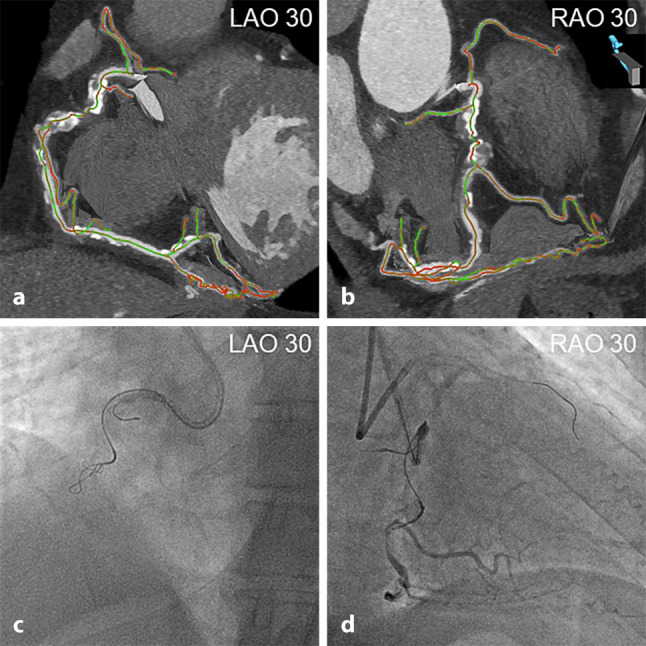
a–d Coronary computed tomography angiography (*CTA*) for guiding the knuckle wire technique. Colour-coded CTA multiplanar reconstructions demonstrating the exact course of a severely calcified and tortuous CTO of the right coronary artery (*RCA*) with clear delineation of the uptake of the right ventricular branch in right anterior oblique (*RAO*) 30° projection. The coronary segments are displayed in *green* (no foreshortening) or *red* (substantial foreshortening) (a, b). Based on CTA reconstructions aligned with the angulation of the C‑arm, the knuckled Fielder XT‑A guidewire (Asahi Intecc, Nagoya, Japan) supported by the Turnpike Spiral microcatheter (Teleflex, Wayne, PA, USA) was intentionally advanced along the course of the RCA, and not into the right ventricular branch (c, d). *LAO* left anterior oblique
